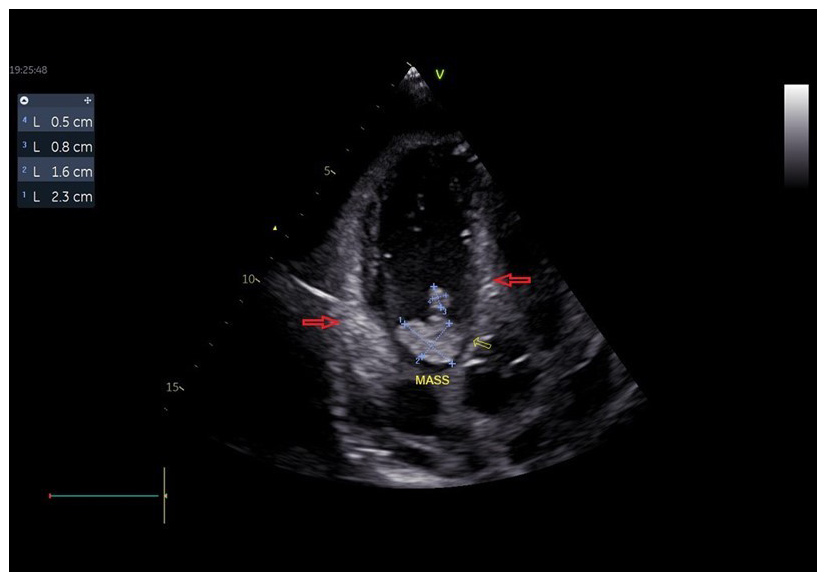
Echocardiography showed the presence of a mass in the right atrium and septal leaflet of tricuspid (yellow arrow). Hyperechoic areas were found in the annulus of tricuspid, lateral wall of right atrium and right ventricle, and interventricular septum (red arrows).
